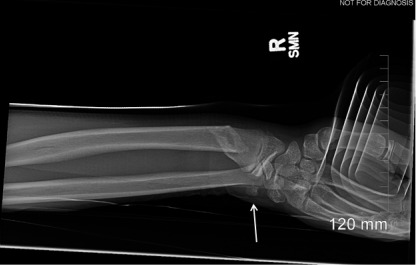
Right wrist radiograph, oblique view, of a child with pisiform bone dislocation. Arrow indicates the dislocated pisiform.
